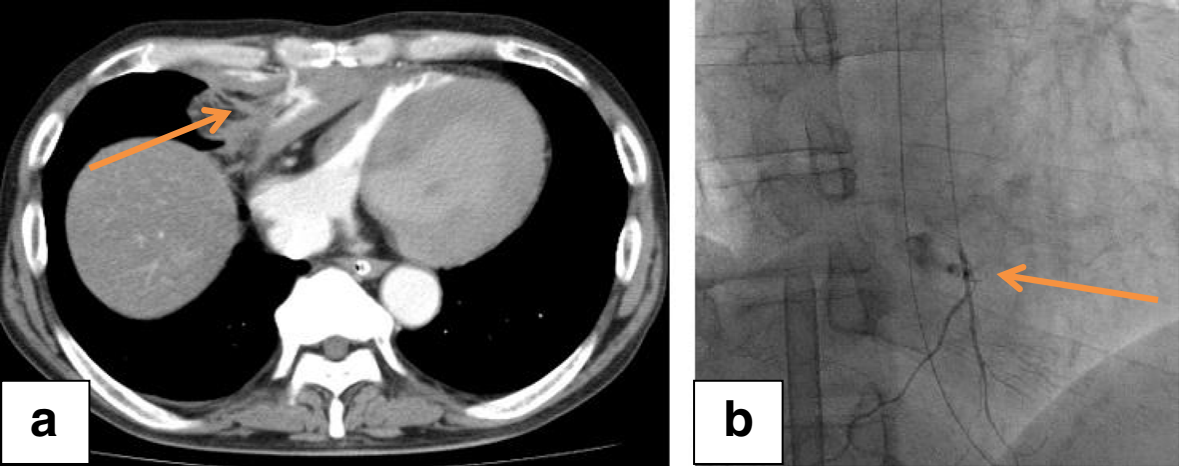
Computed tomography and angiography findings from Patient 5. a Contrast-enhanced computed tomography from before the coronary angiography revealed right rib fractures, a sternum fracture, and a hematoma at the mediastinum with contrast extravasation (*arrow*). b Angiography after the coronary angiography revealed contrast extravasation at the right internal mammary artery (*arrow*)
